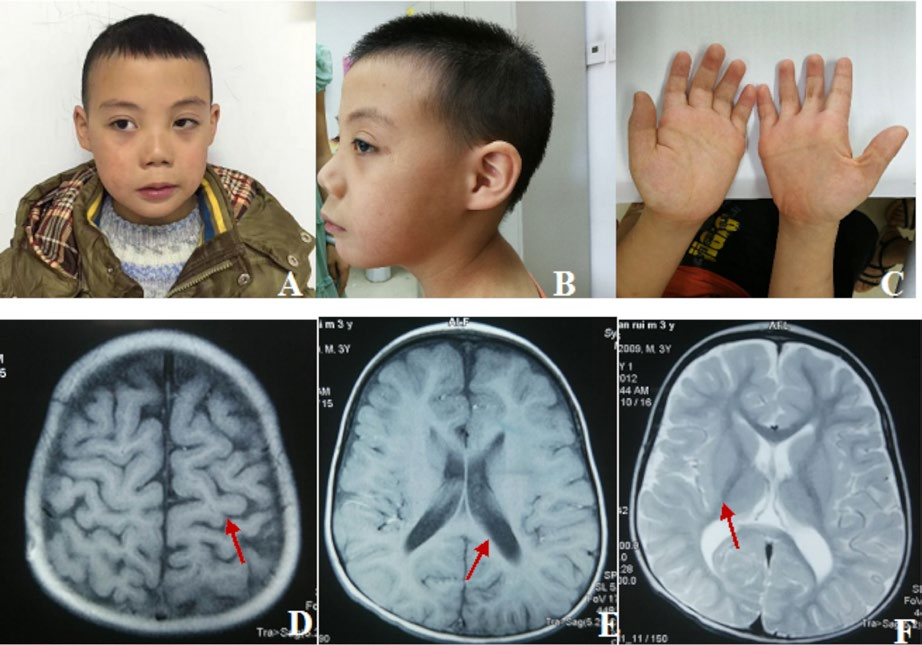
Clinical features of the case 1. (a, b) He had dysmorphic features including hypertelorism, a broad forehead, long philtrum, upslanting palpebral fissures, hypoplastic columella and ala nasi, thin upper lip, high‐arched palate, epicanthic fold, and micrognathia. (c) He had brachydactyly and a simian crease on his right hand. (d–f) Brain magnetic resonance imaging (MRI) scans showed the sulci and the lateral ventricle widened, the white matter volume was less than normal (arrows)
